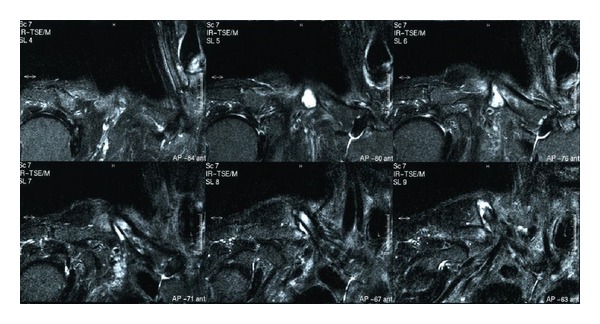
Preoperative MRI STIR images demonstrating a formation of pseudoarthrosis with fluid collection and suggesting bone marrow edema at both ends of the fracture fragments.
